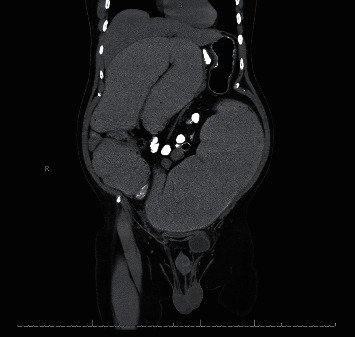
Coronal section of computed tomography without contrast of the abdomen and pelvis demonstrating significant dilation of the colon proximal to the colonic anastomosis from prior sigmoidectomy consistent with an obstructing anastomotic stricture.
